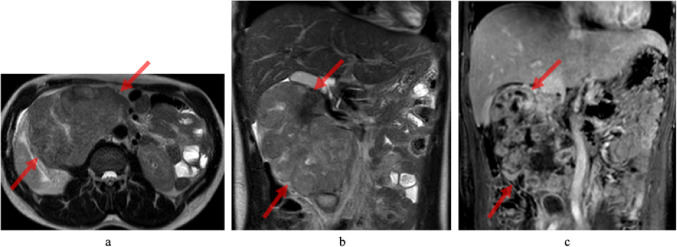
Axial and coronal T2-weighted images (a, b) without fat saturation and a coronal fat-saturated T1-weighted contrast-enhanced image (c) demonstrate a large heterogeneously enhancing right retroperitoneal mass (red arrows). The patient had biopsy proven leiomyosarcoma arising from the IVC
